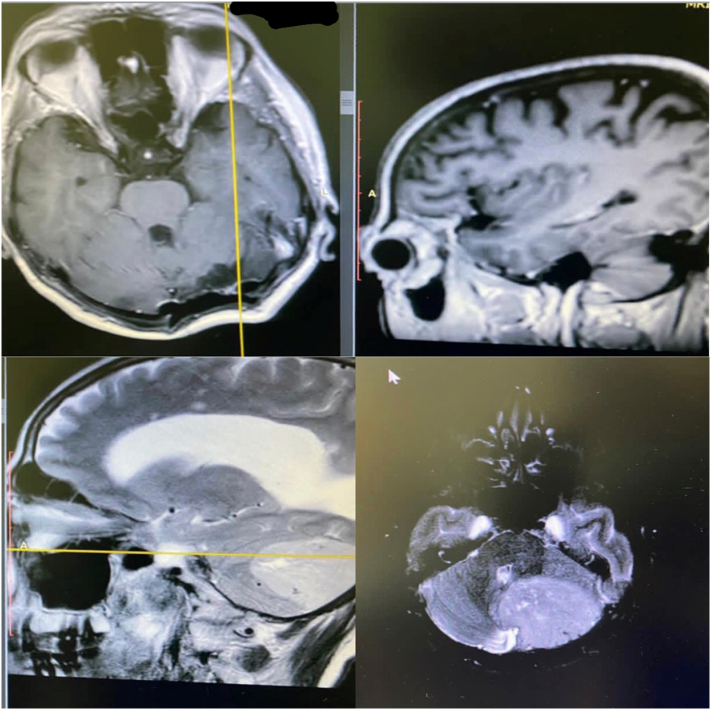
Brain MRI showing a left cerebellar mass of unknown origin which was further analyzed pathologically giving us a diagnosis of liponeurocytoma.
